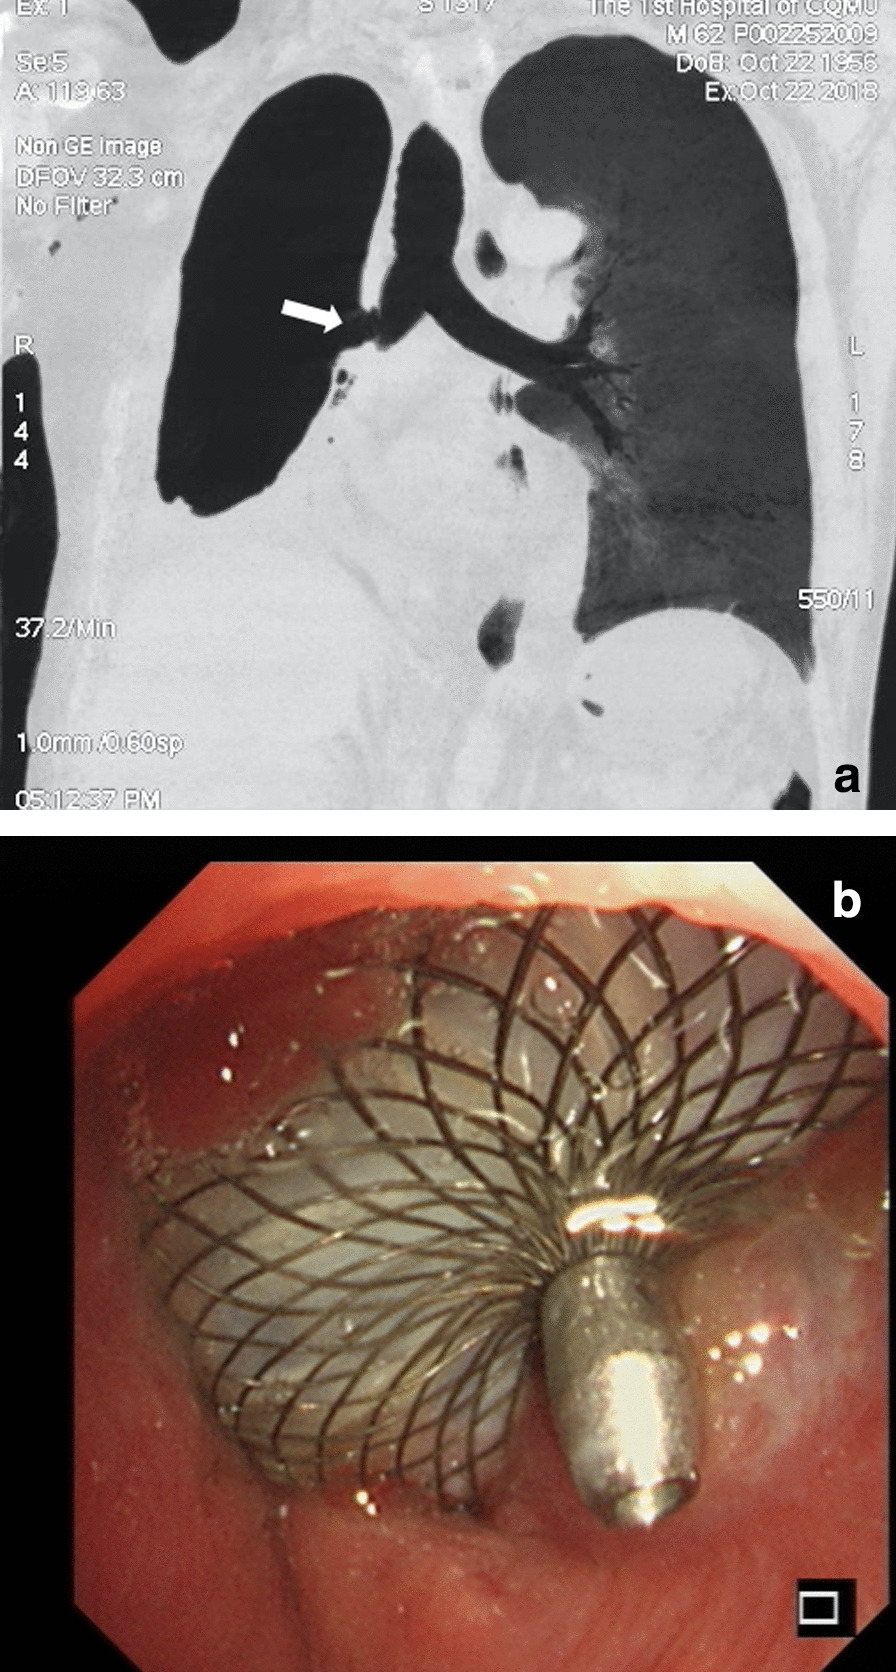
Patient no. 4 in Table 1. a Measuring the size of the BPF (white arrow) localized in the right middle bronchus via three-dimensional reconstruction of chest computed tomography images. b The bronchoscopic view of the large central BPF located in the right main bronchus completely closed by the VSD occluder with surrounding granulation tissue
